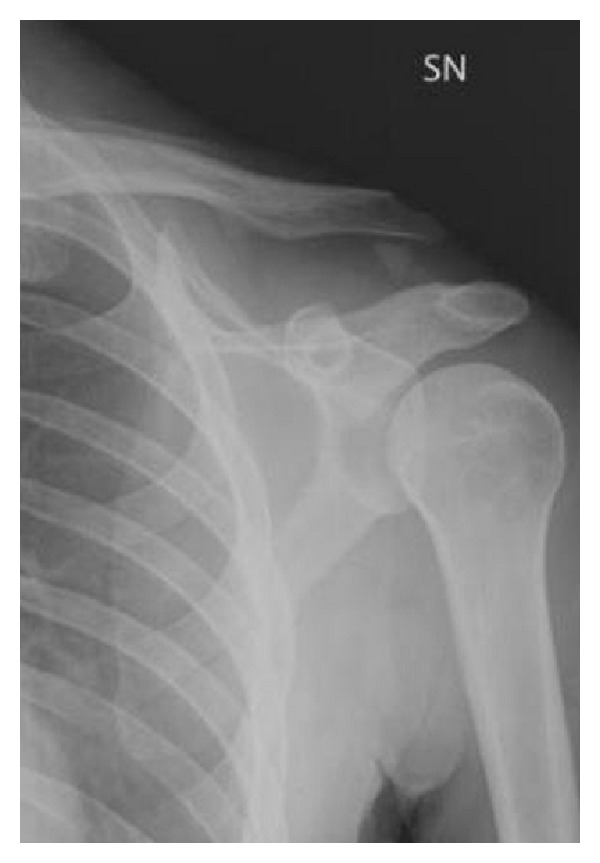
Radiograph (AP view) of a 34-year-old male showing an ACJ dislocation complicated with a fracture of the lateral third of the clavicle.
